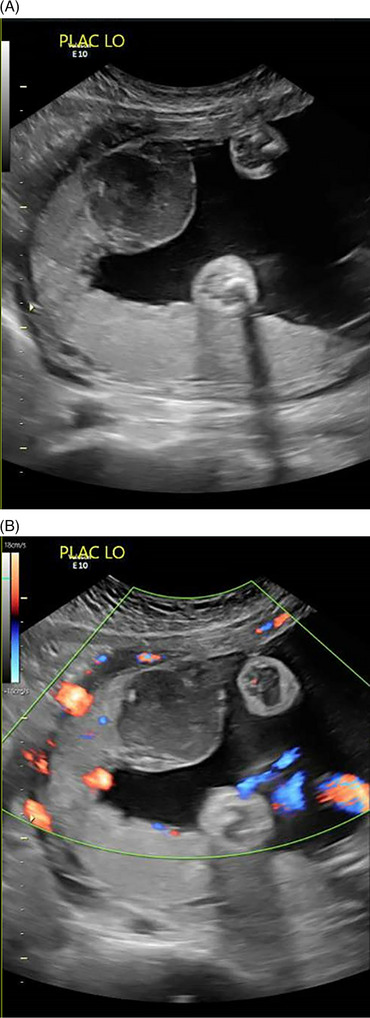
(A) Sonographic appearance of giant chorangioma with homogeneous echotexture. (B) Color Doppler interrogation demonstrates the absence of vascular flow within the tumor, consistent with spontaneous thrombosis.
